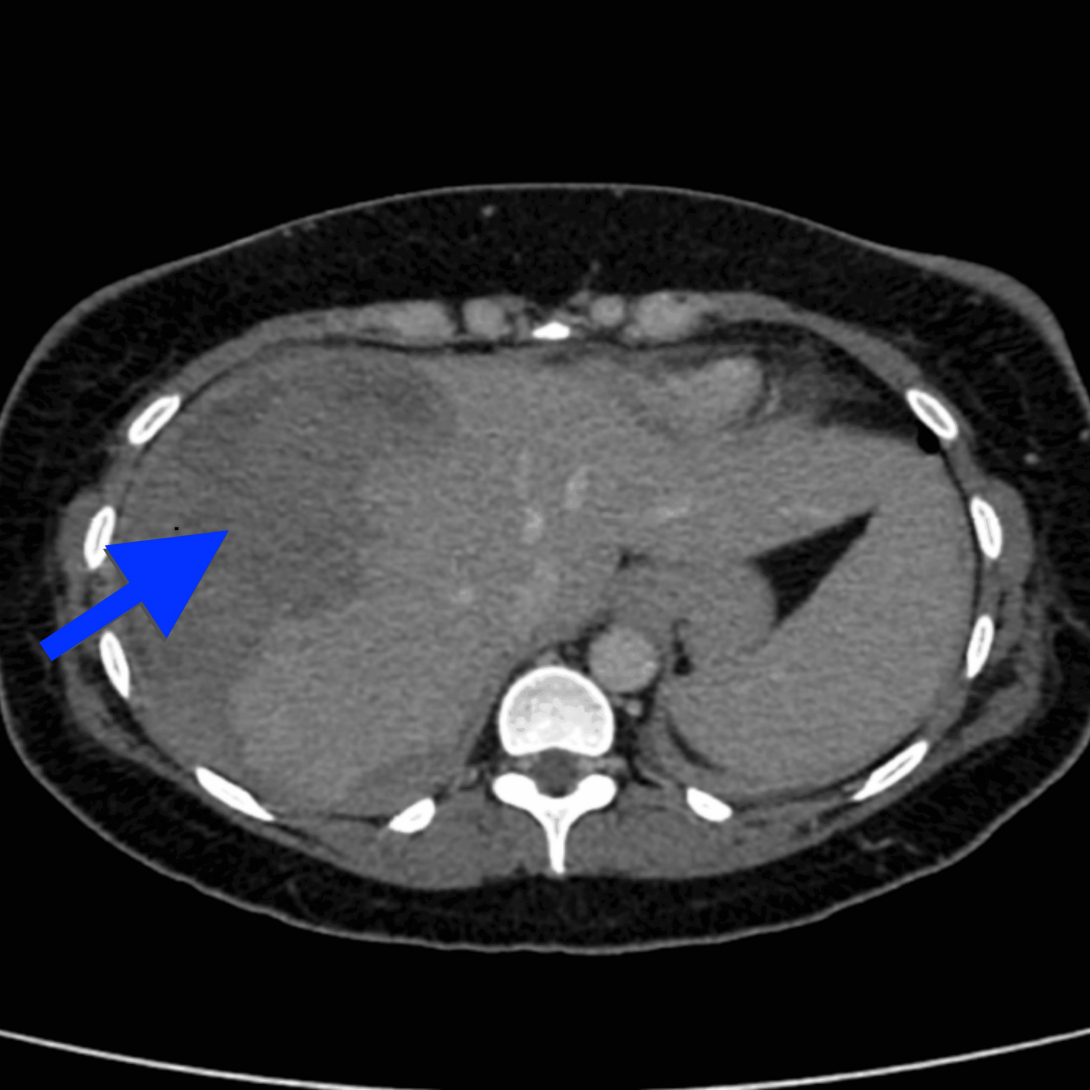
Axial section of the CT scan performed on day one showing a large subcapsular hepatic haematoma
